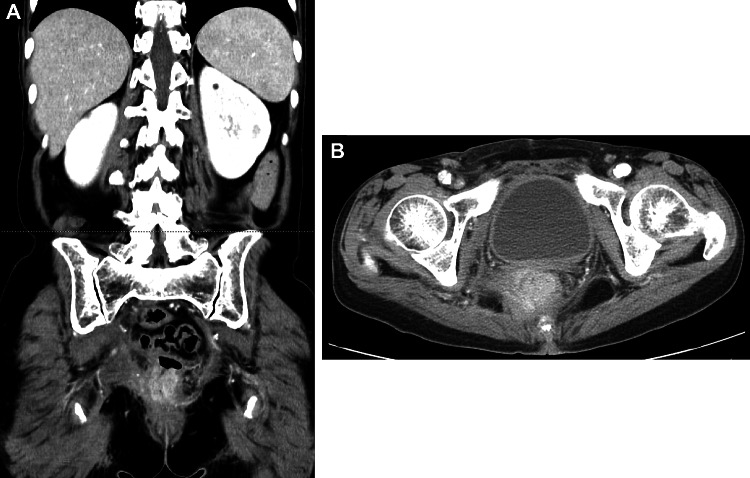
Abdomino-pelvic CT scan after chemotherapy. A marked shrinkage of rectal tumor was demonstrated
